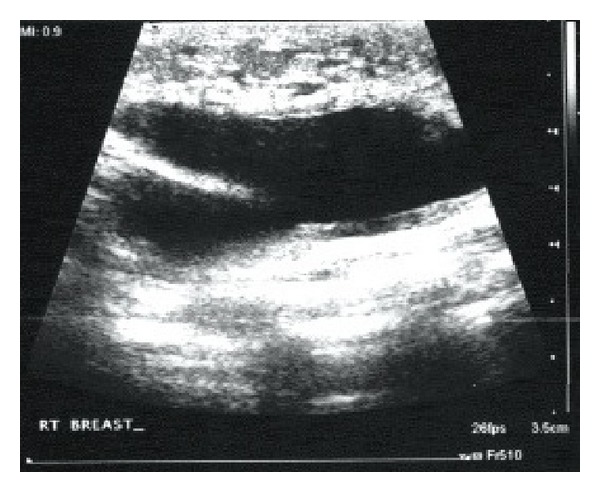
Ultrasound of the chest/axilla showing a cystic mass with septations in the right lateral chest wall near the right axilla, 5 cm × 4 cm.
